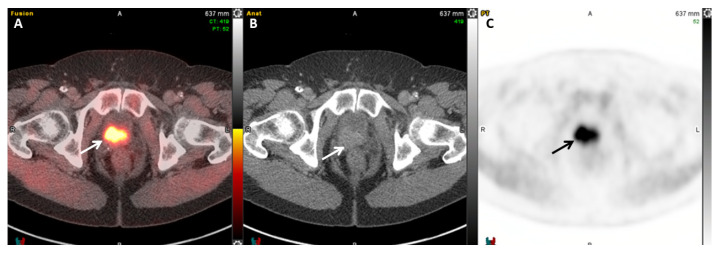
An 84-year-old man with biochemically recurrent prostate cancer 4 years following radical prostatectomy. PSA was 5.2 ng/mL at the time of PET/CT imaging. Fused axial PET/CT (A), axial CT (B), and PET (C), ^18^F-fluciclovine images demonstrate an area of increased radiotracer uptake (SUVmax = 13.7) corresponding to a 3.6 × 2.6 cm enhancing lesion at the prostatectomy bed invading the bladder neck and posterior wall of the urinary bladder (arrows).
